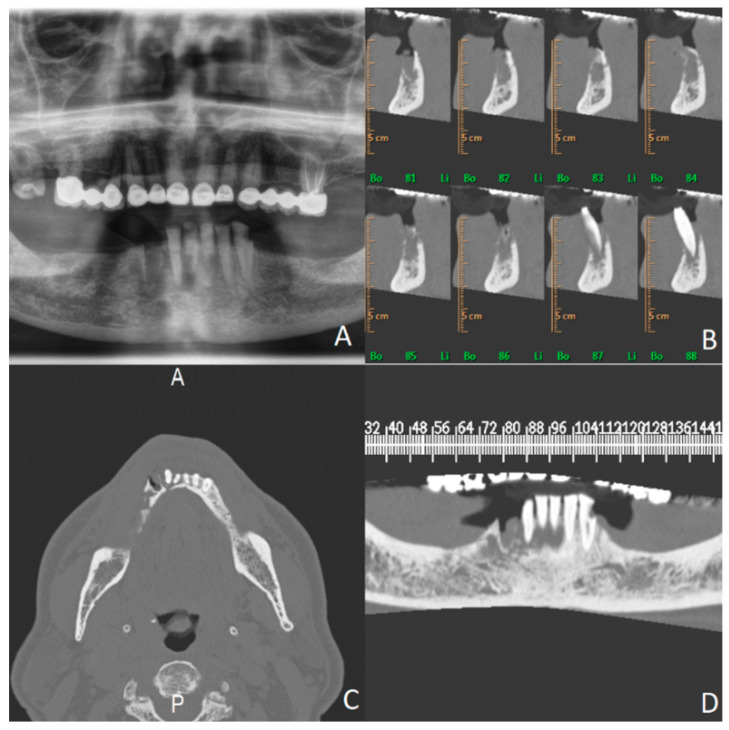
Preoperative radiographs. (A) Preoperative panoramic view. (B) Pre-operative computed tomography sagittal view. (C) Pre-operative computed tomography axial view. (D) Pre-operative computed tomography frontal view.
